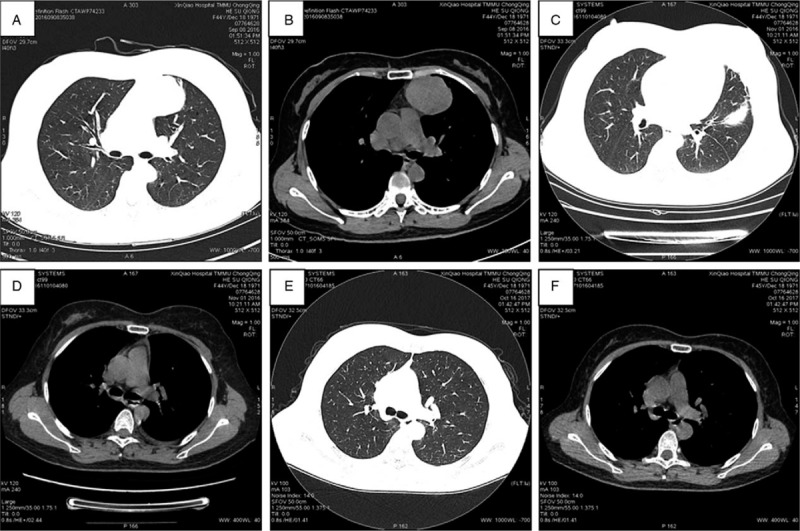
Preoperative chest computed tomography (CT) shows a mass in the left anterior mediastinum (A and B); chest CT at postoperative month 1 shows pulmonary infection in the left upper lobe (C) and a small amount of pleural fluid on the left side (D); and chest CT at postoperative month 6 shows normal lungs and no pulmonary fibrosis in the irradiated region (E and F).
